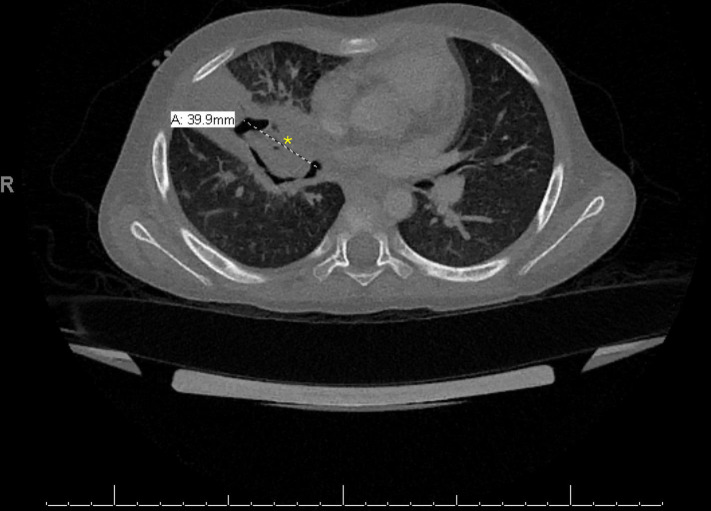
CT chest reveals a large cavity in the perihilar region with surrounding consolidation and a central soft tissue filling defect. Asterisk overlies cavity located in the right middle lobe, near the interlobar artery stump (approximately 7 mm).
